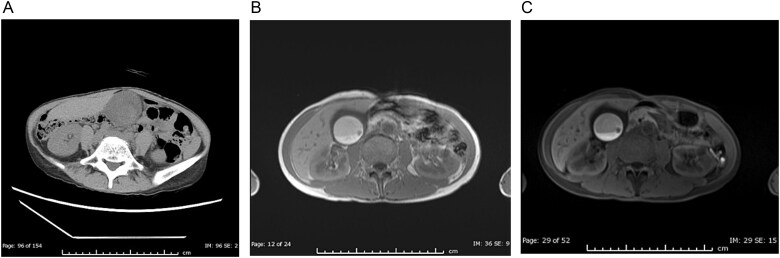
Imaging findings at emergency presentation. (A) Non-contrast CT showing a markedly distended gallbladder filled with high-density material (92 Hounsfield units), indicating acute intracholecystic hemorrhage. (B) T1-weighted MRI revealing high signal intensity within the gallbladder, consistent with a hematoma. The previously noted polypoid lesion is obscured by the haemorrhage. (C) T1-weighted MRI with fat suppression, the high-signal area within the gallbladder is not suppressed.
